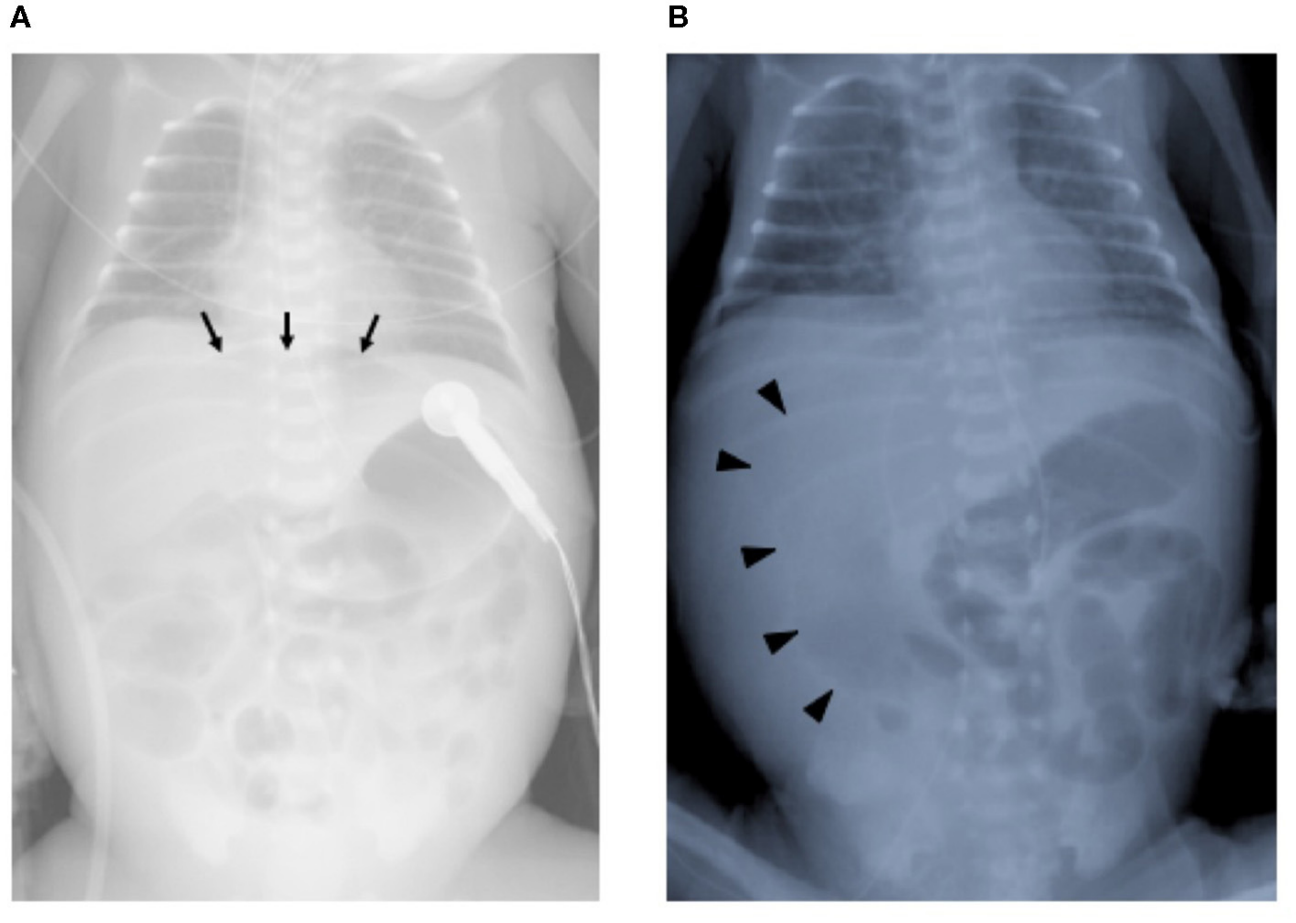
Chest and abdominal radiographs at the ileal perforations. (A) First perforation at day of life 8. Arrows indicate free air in the intraperitoneal space. (B) Second perforation at day of life 17. Arrowheads indicate free air in the intraperitoneal space.
